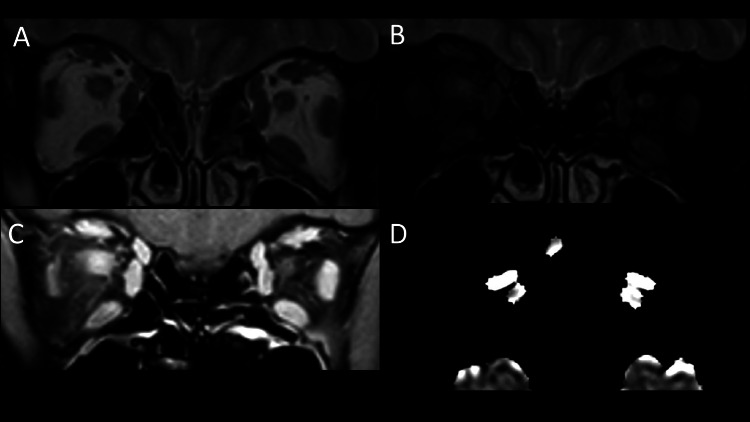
Normal extraocular muscles. Coronal T2-weighted MRI (A) demonstrating no muscle enlargement and no hyperintensity within the muscles.Coronal fat-suppressed T2-weighted MRI (B) showing symmetrical signal intensity of the muscles. Fat-suppressed contrast-enhanced T1-weighted MRI (C) showing symmetrical enhancement of the extraocular muscles. ADC map (D) showing no hyperintense signals within either orbit
